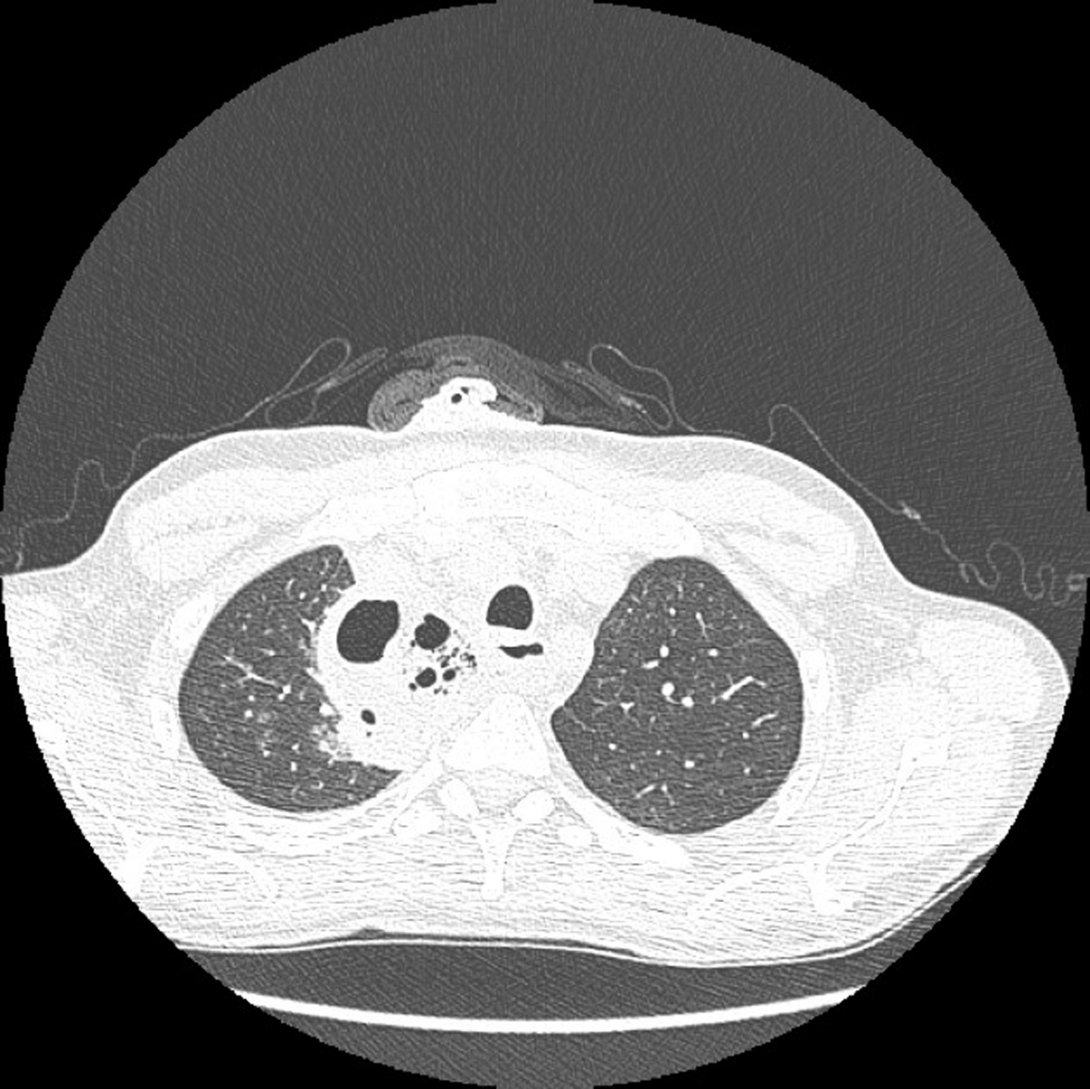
CT scan demonstrated soft-tissue swelling of the neck and encapsulated fluid collections with gas bubbles within anterior mediastinum, especially on the right side.
